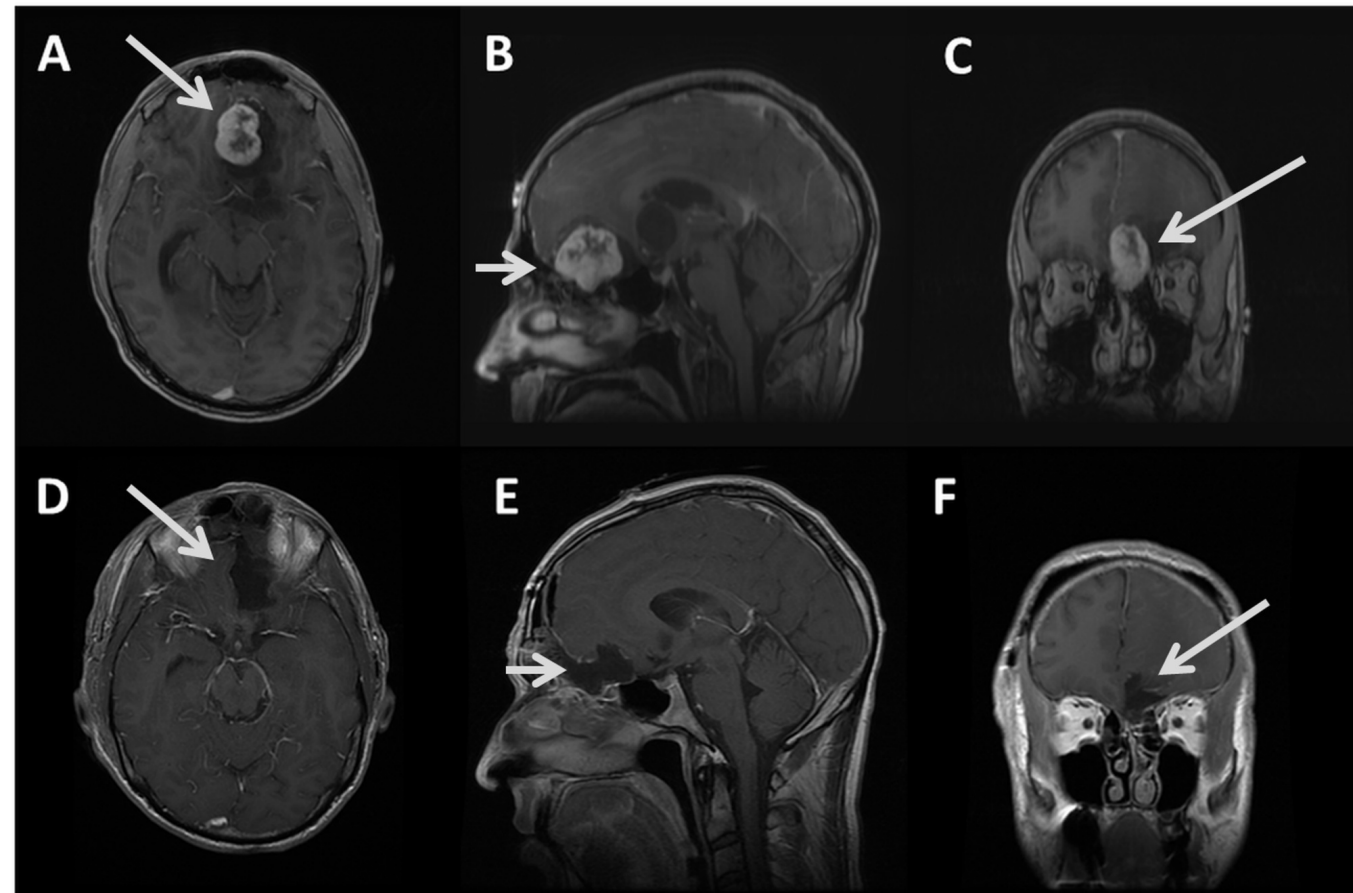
Pre- and postoperative brain magnetic resonance imaging (MRI) demonstrating gross total resection of nasoethmoid schwannoma with intracranial extension Preoperative axial (A) sagittal (B) and coronal (C) gadolinium enhanced MRI demonstrating cystic and heterogeneously enhancing solid mass (arrows) extending from the mid-left ethmoid air cells into the anterior cranial fossa, left frontal lobe, and left basal ganglia, causing mass effect on the left lateral ventricle and midline shift. Postoperative axial (C) sagittal (D) and coronal (E) gadolinium enhanced MRI demonstrating gross total resection (arrows demonstrating resection cavity with no residual tumor).
